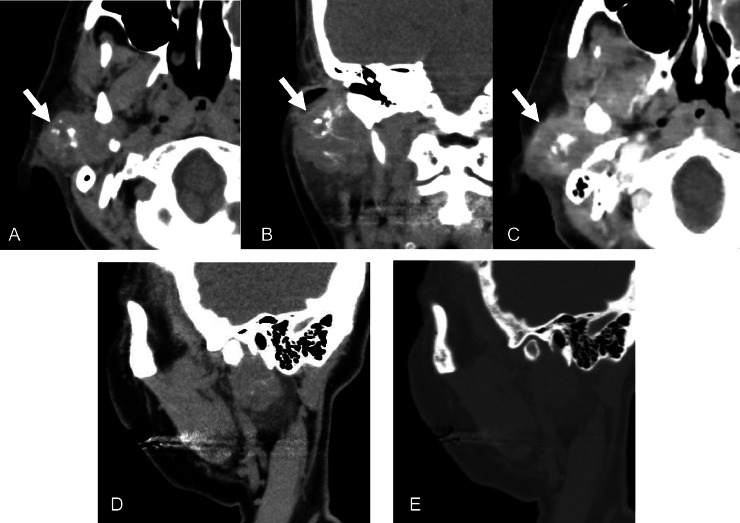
(A and B) Axial and coronal computed tomography revealed a lobulated mass that displaced the right parotid gland and extended into the parapharyngeal space. The boundary of the mass was partially obscured. The lesion exhibited coarse internal calcification. (C) On axial enhanced computed tomography, the mass exhibited heterogeneous contrast enhancement with marginal predominance. (D and E) Sagittal computed tomography revealed no apparent destruction or infiltration of the surrounding bone tissue.
